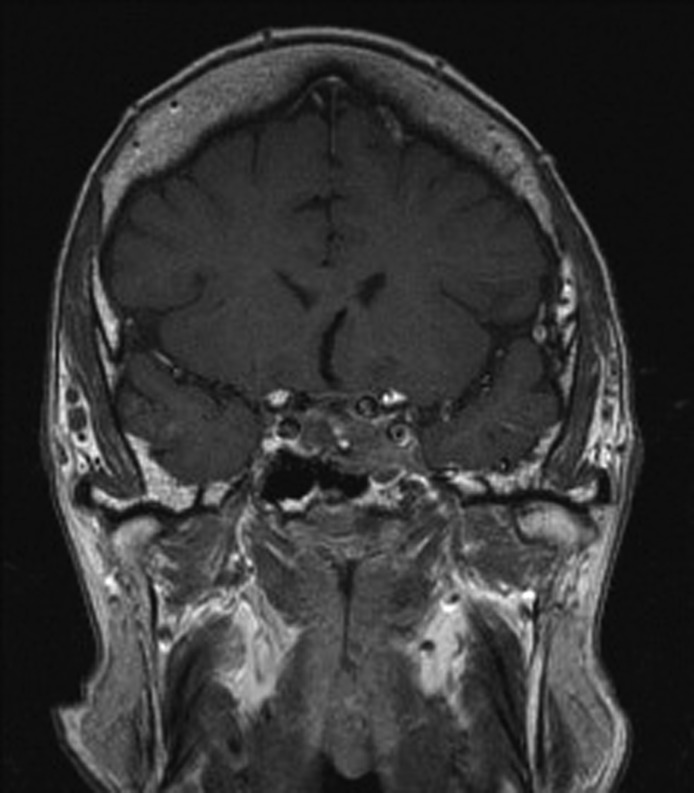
Three months after pasireotide therapy. MRI scan of pituitary – coronal view – T1 weighted image with gadolinium contrast showing residual pituitary tumour with invasion of cavernous sinus showing loss of enhancement.
